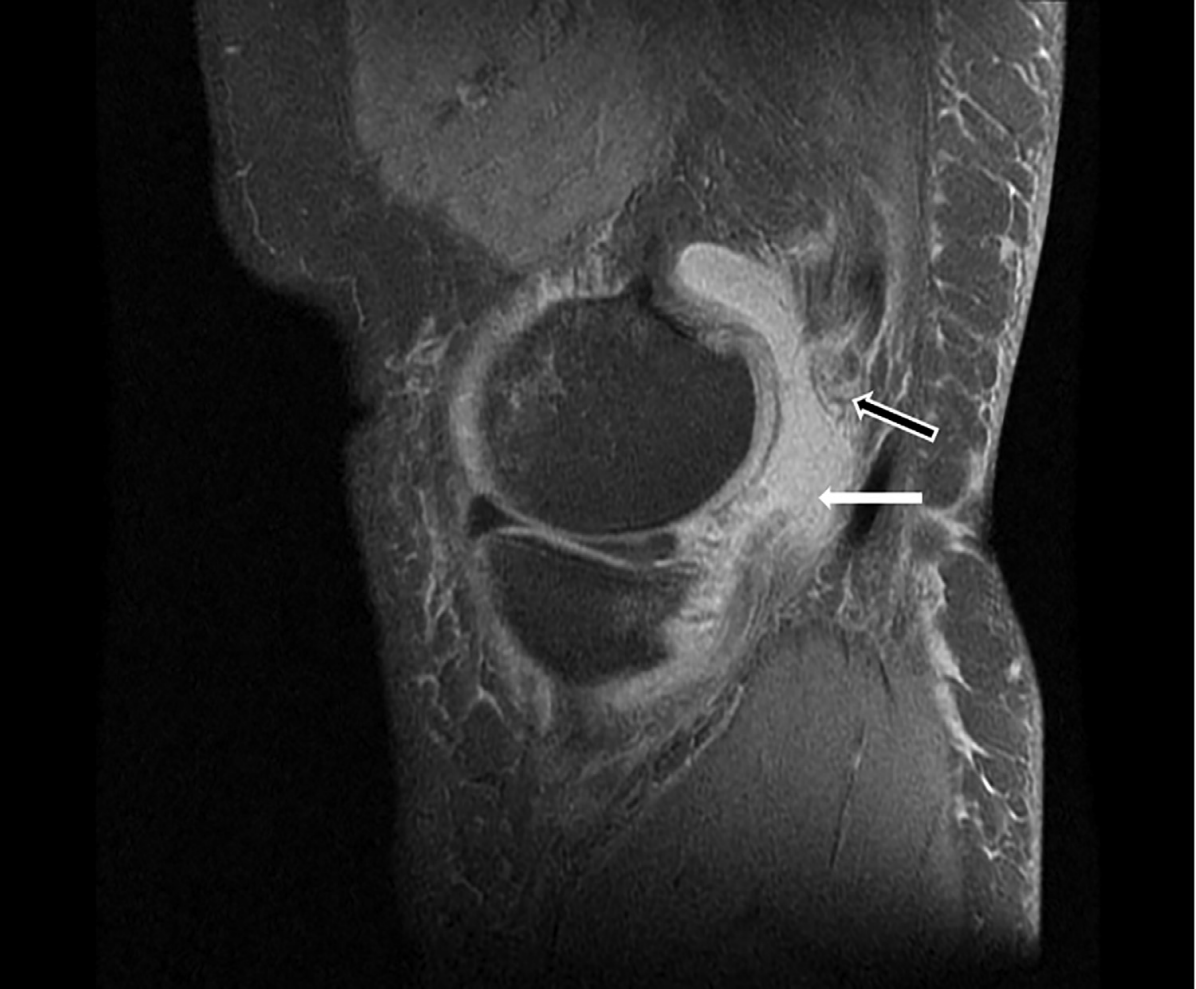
T2-weighted sagittal image at presentation. The black arrow demonstrates the retracted distal end of the semimembranosus tendon. The white arrow demonstrates the posterior capsular tear with fluid filled space secondary to the rupture.
